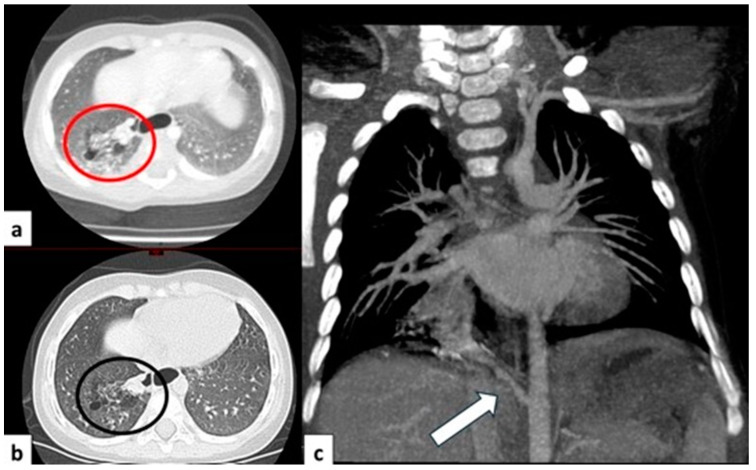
CT axial image visualized on lung window (a) of a 9 month old boy with right cystic lesions (previously seen on fetal ultrasound) shows an area of parenchymal consolidation mixed with air-filled cystic lesions, located in the right lower lobe (red circle). Contrast-enhanced acquisitions (c) demonstrate an abnormal arterial supply from systemic circulation into sequestered lung (arrow). These findings are compatible with hybrid lesion (CPAM and pulmonary sequestration). A CT scan performed after the arterial embolization of the aberrant vessel (b) shows a mild reduction in sequestered lung parenchyma (black circle).
